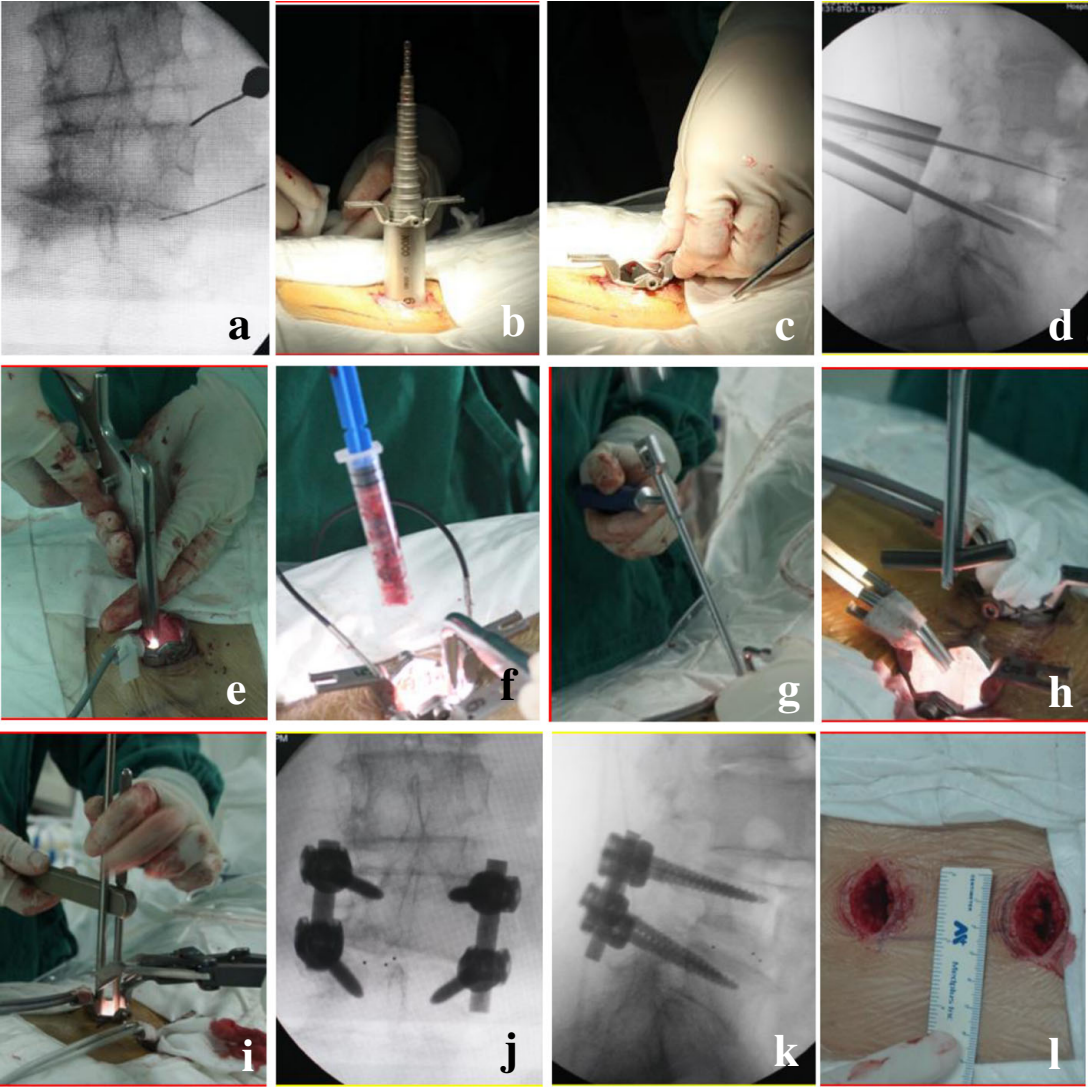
The MIS-TLIF technique. a Position the level under the C-arm fluoroscopy. b, c The tubular retractor was placed. d The road of pedicle screw was prepared. e The progress of decompression was performed. f, g Bone and cage interbody graft was inserted. h, i: The progress of compression was performed. j, k The postoperative X-ray showed good result. l The incision was about 2.5 cm
